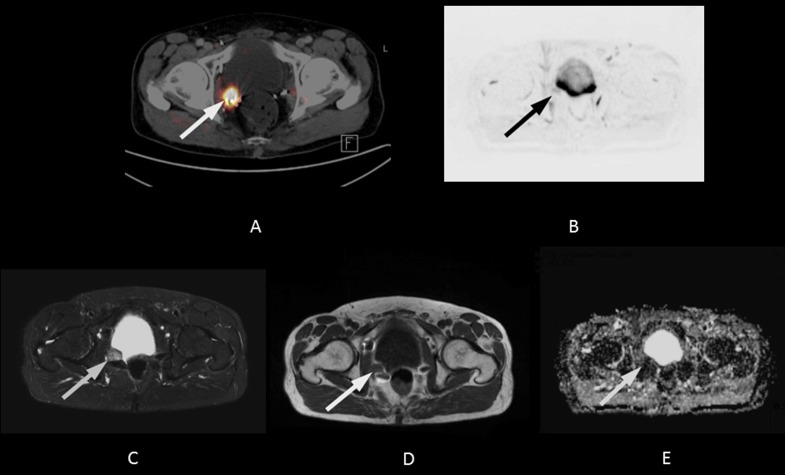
68-year-old patient with local recurrence 13 years after radical prostatectomy (initial Gleason Score: 8) The PSA level at the time of imaging was 2.1 ng/mL. A fall in PSA level after pelvic irradiation confirmed the lesion was malignant (< 1.0 ng/mL after radiotherapy). A. The axial PET/CT fusion image shows focal 11C-choline uptake in the area of the right neurovascular bundle (white arrow, SUV max 4.2). B. The lesion was not visible on the 500 ms b-image (B) or the ADC map (not shown) of the DWI. Both the STIR image C. and the T1w image D. show susceptibility artefacts due to surgical clips in the area of focal 11C-choline uptake. Part of the lesion, however, remains visible in spite of some signal loss (white arrows).
